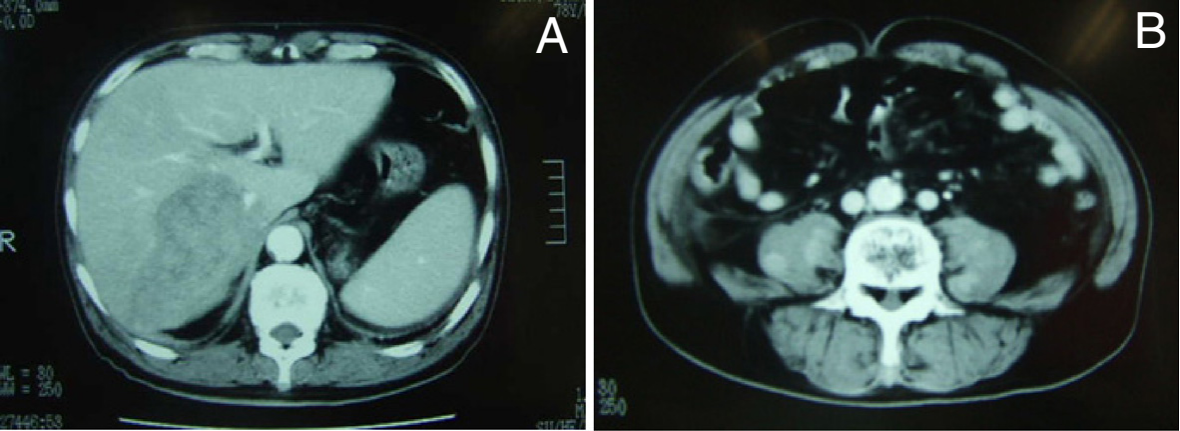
The findings of an abdominal CT scan. (A) An ill-defined hepatic mass approximately 9 cm in diameter occupied the right lobe. (B) Multiple enhanced nodules in the bilateral iliopsoas muscles were noted.
